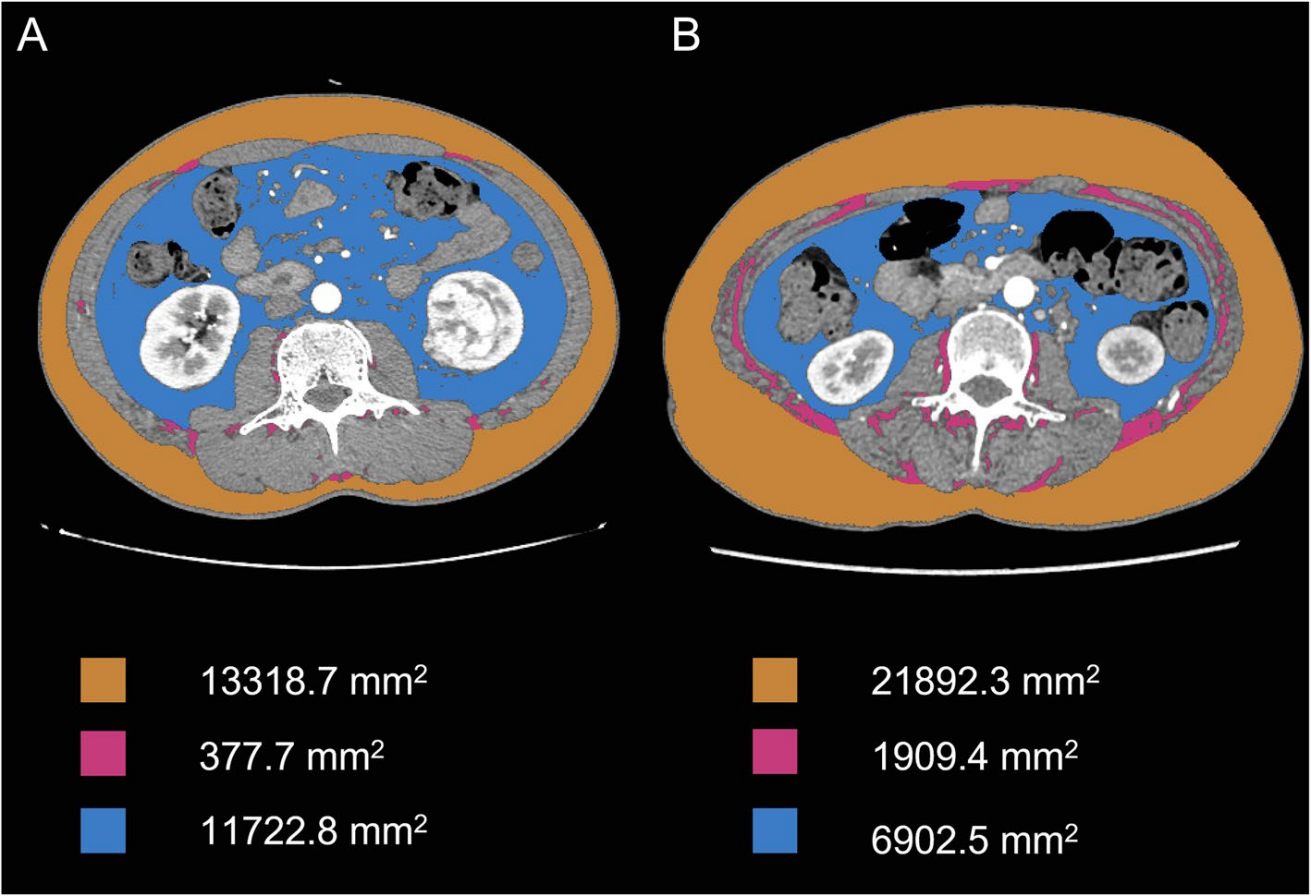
Representative cross-sectional CT images and fat area of each part. A Image of a 42-year-old man with WHO/ISUP II ccRCC. B Image of a 60-year-old woman with WHO/ISUP IV ccRCC. The subcutaneous adipose tissue area (orange), intramuscular adipose tissue area (red), and visceral adipose tissue area (blue) were quantified
